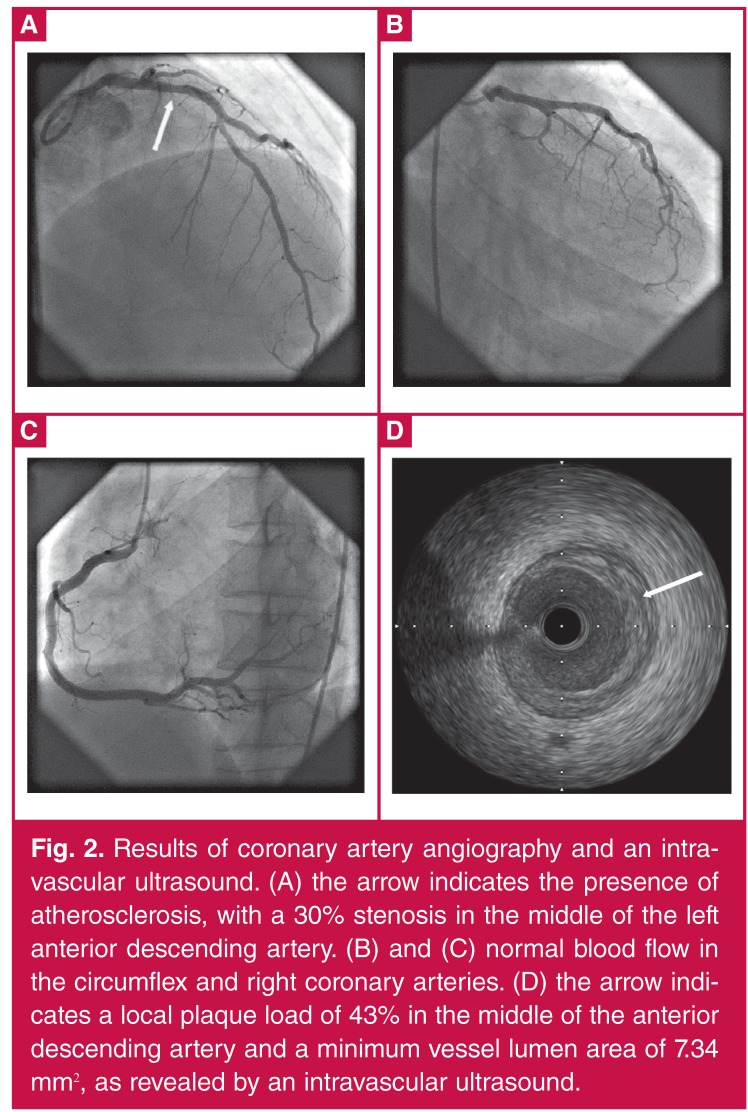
Results of coronary artery angiography and an intravascular ultrasound. (A) the arrow indicates the presence of atherosclerosis, with a 30% stenosis in the middle of the left anterior descending artery. (B) and (C) normal blood flow in the circumflex and right coronary arteries. (D) the arrow indicates a local plaque load of 43% in the middle of the anterior descending artery and a minimum vessel lumen area of 7.34 mm^2^, as revealed by an intravascular ultrasound.
